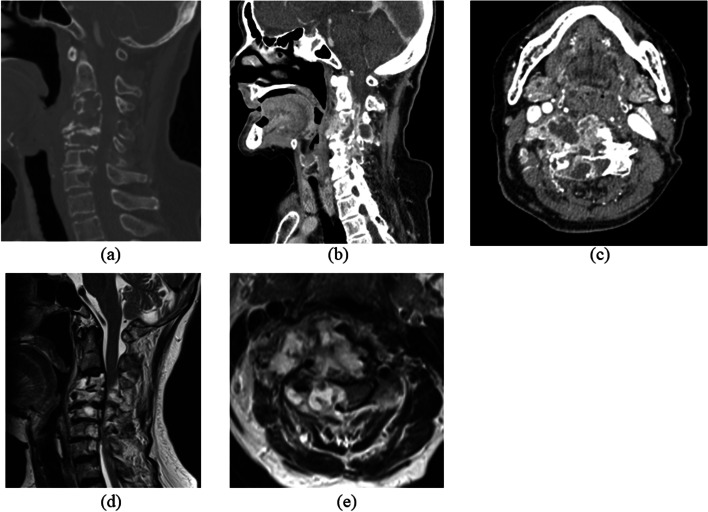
Preoperative CT and MRI. a-c Cervical contrast CT revealed osteolytic lesions in C3–7. The masses extended into the spinal canal from within the vertebrae, compressing the cervical spinal cord from the right side. d, e MRI T2-weighted imaging showed a mass with the same signal as bone from within the C3–7 vertebrae to outside the vertebral bodies. The cervical spinal cord at the same levels was compressed from the right side
